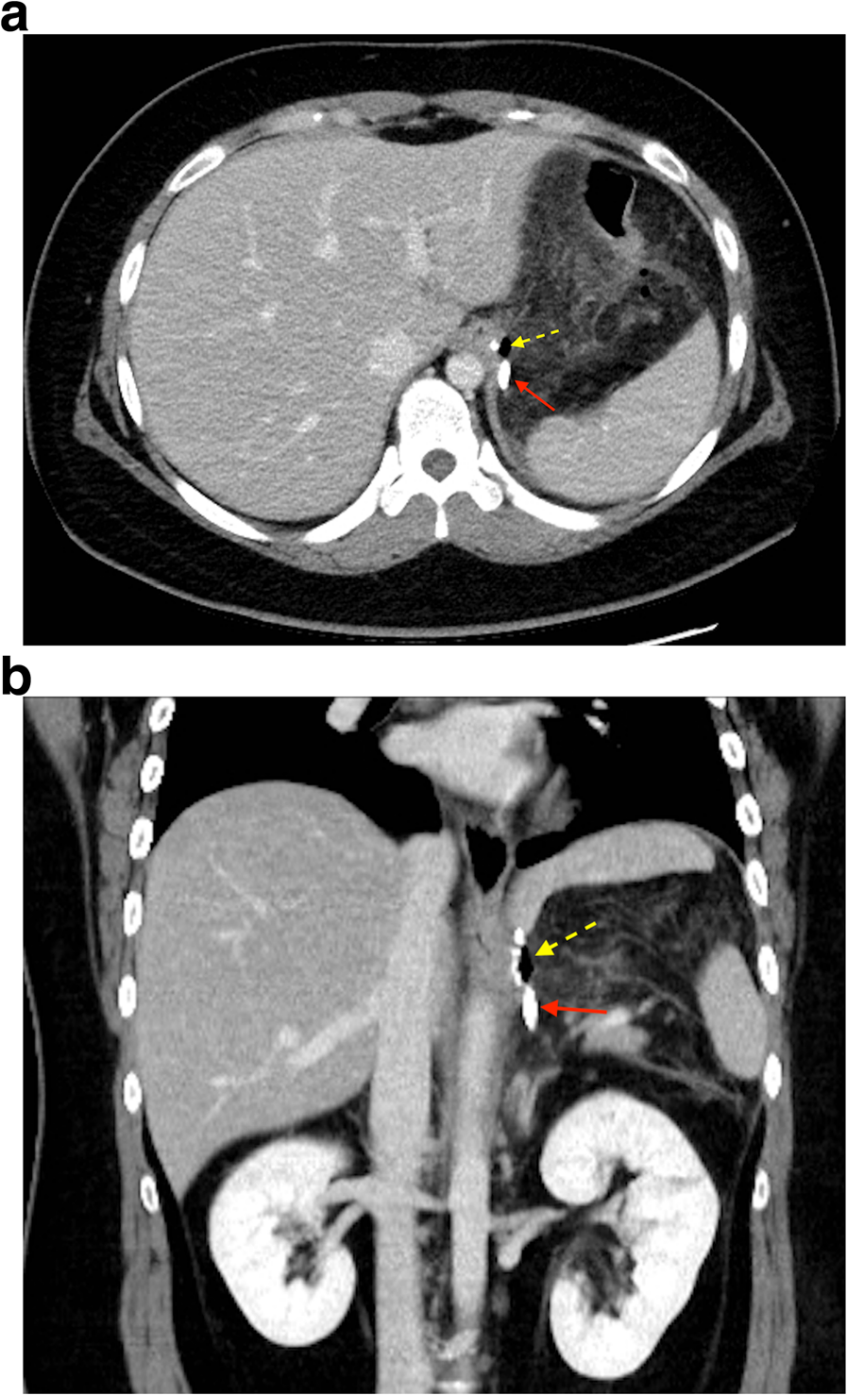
CT scan, axial (a) and coronal (b) views, at POD7. The minor leak with a small air-pocket was denoted by the dashed arrow, and there was some fat-stranding nearby but no obvious fluid accumulation. The solid arrow indicated the tip of the CWV drain placed intraoperatively. Abbreviation: POD, postoperative day; CT, computed tomography; CWV, closed wound vacuum
